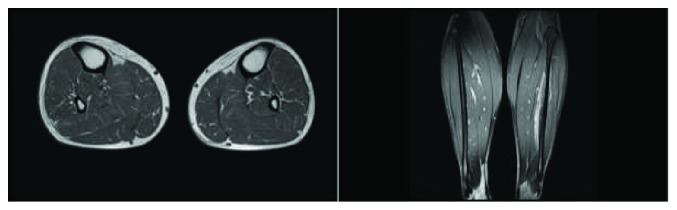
MRI of the left leg showing patchy increased STIR signal in the muscles but predominately involving the gastrocnemius muscles.
